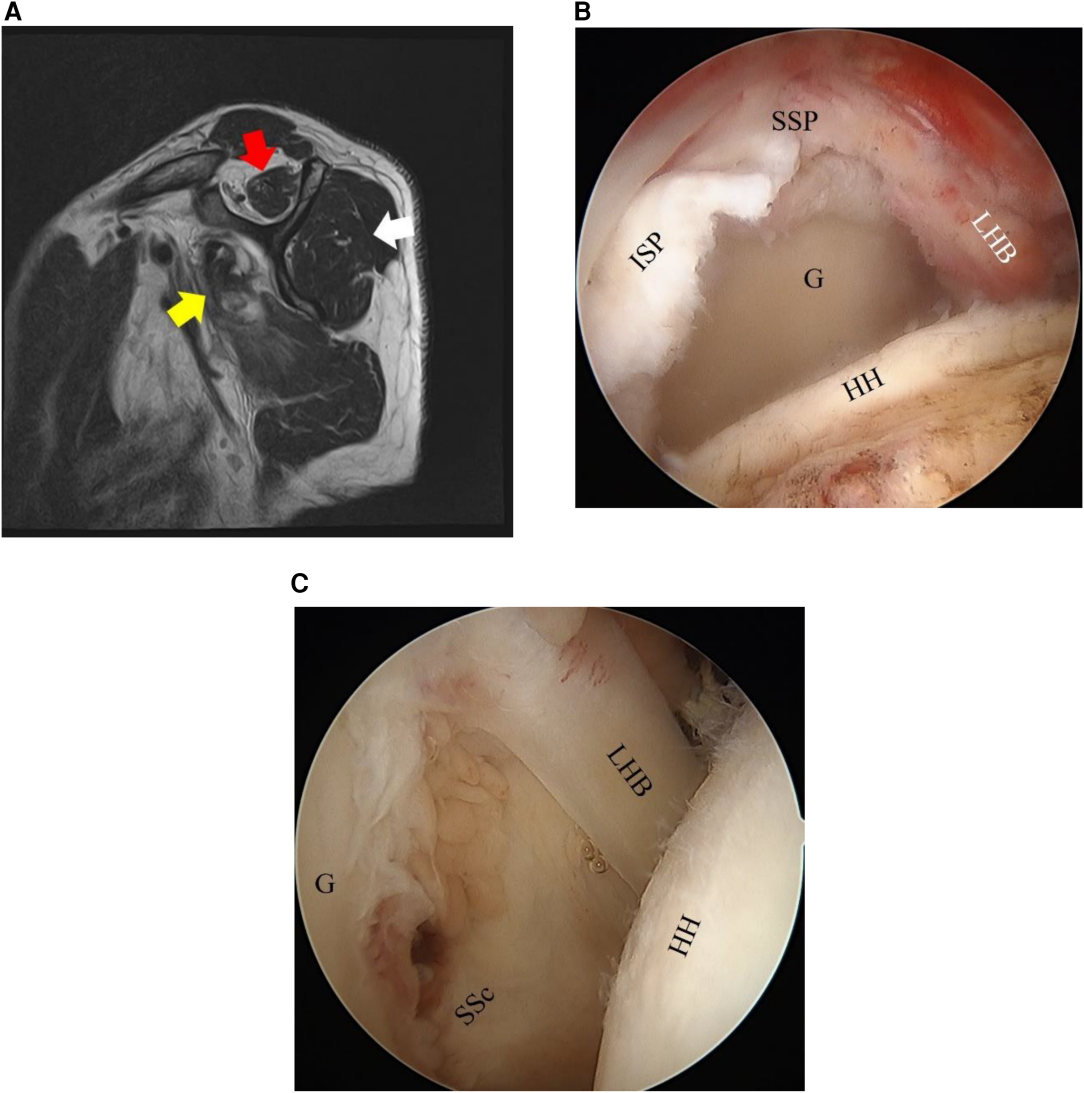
Fatty infiltration (FI) of the rotator cuff muscles on MRI sagittal images and arthroscopic findings of Case 1. (A) A 64-year-old man. Right rotator cuff tear. The FI grades of the SSP (red arrow), ISP (white arrow), and SSc (yellow arrow) are 2, 1, and 2, respectively. (B,C) Based on the arthroscopic findings, the SSP–ISP tear is graded as a massive tear and the SSc tear as Lafosse Type IV. SSP, supraspinatus tendon; ISP, infraspinatus tendon; SSc, subscapularis tendon; G, glenoid; LHB, long head of biceps; HH, humeral head.
